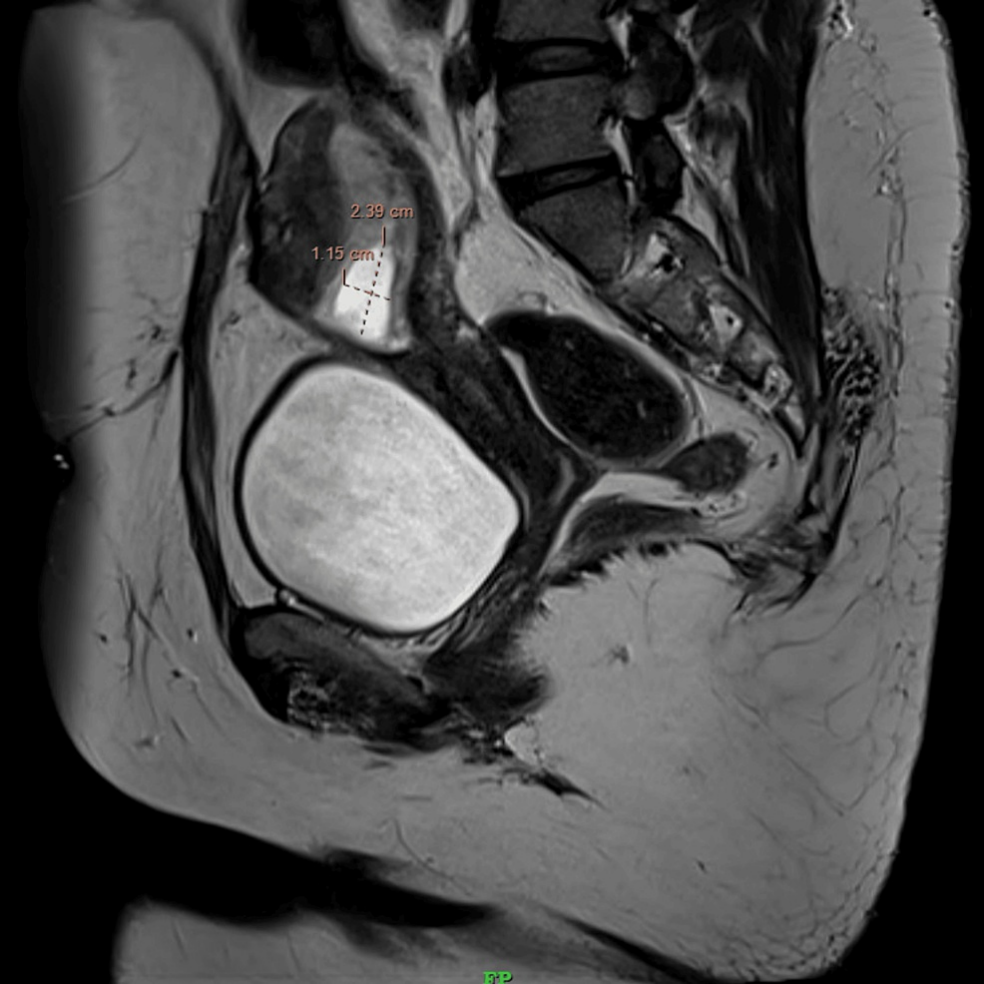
T2-weighted sagittal magnetic resonance imaging showing the gestational sac measuring approximately 1.15 cm × 2.39 cm.
